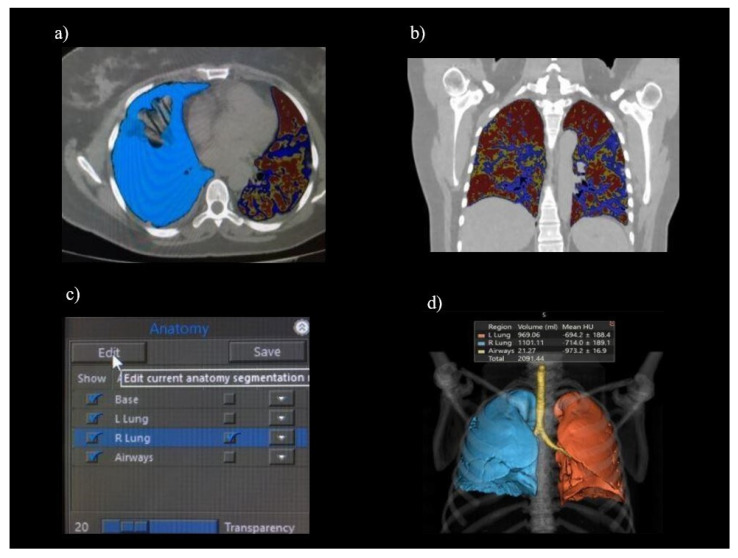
CT Lung Density Analysis. This image shows: (a) parenchyma contouring; (b) lesion display; (c) manual editing; (d) final result with a volumetric rendering.
